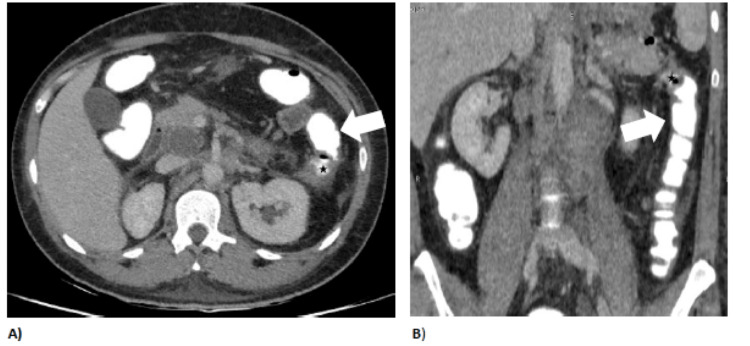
CT with IV and rectal contrast after colonoscopy and before initiation of treatment. Axial (A) and coronal (B) CT abdomen study with IV contrast after colonoscopy showing significant volume reduction and evacuation with air-fluid level of the necrotic lymphadenopathy (asterisk) seen intimately related to the most proximal descending colon (thick white arrow). The rest of the necrotic lymphadenopathy are unchanged. IV, intravenous
